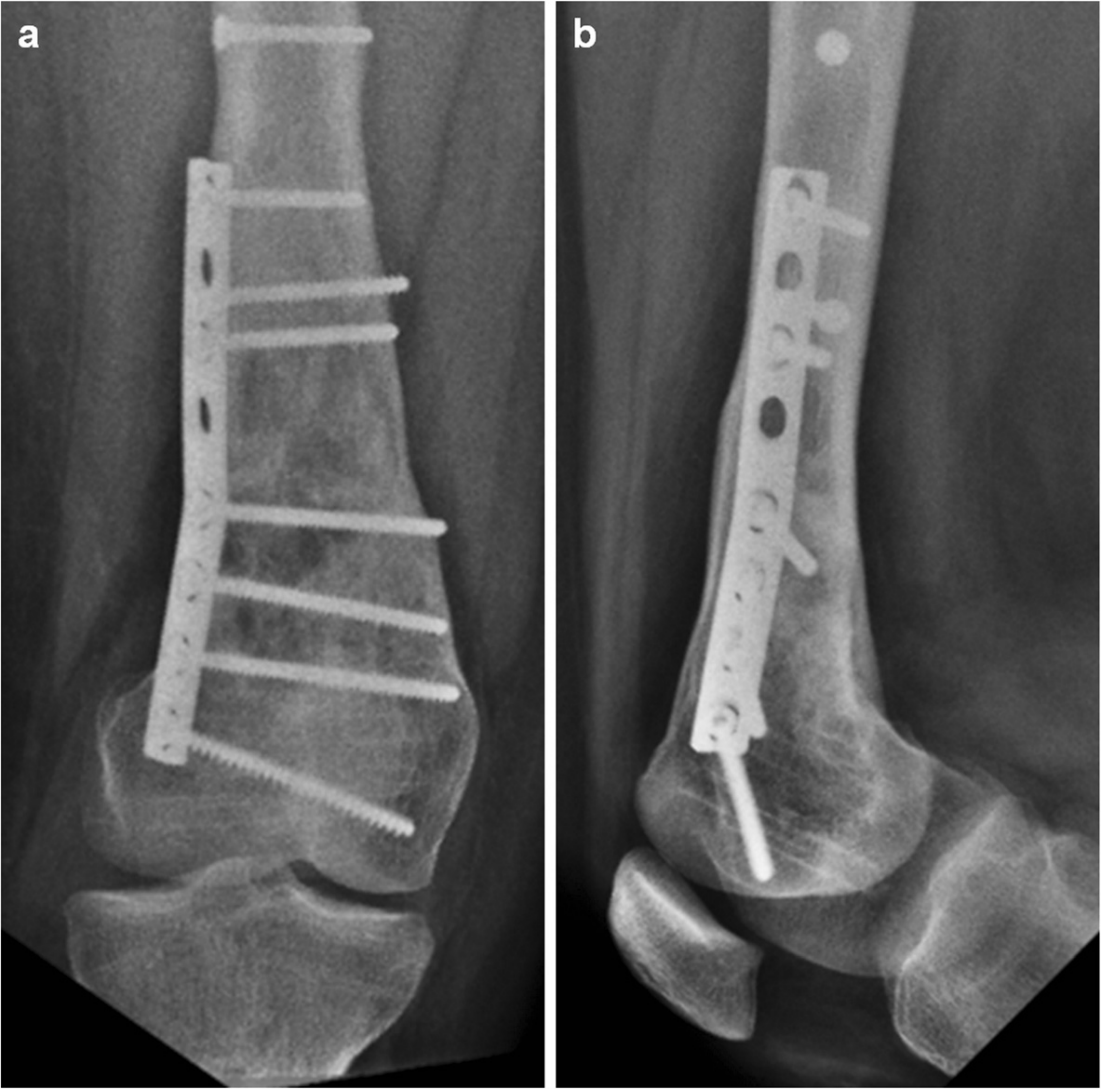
Anteroposterior (a) and lateral (b) radiographs performed 6 months after plate removal, curettage, bone grafting of the remaining NOFs and prophylactic fixation of the distal femur.
